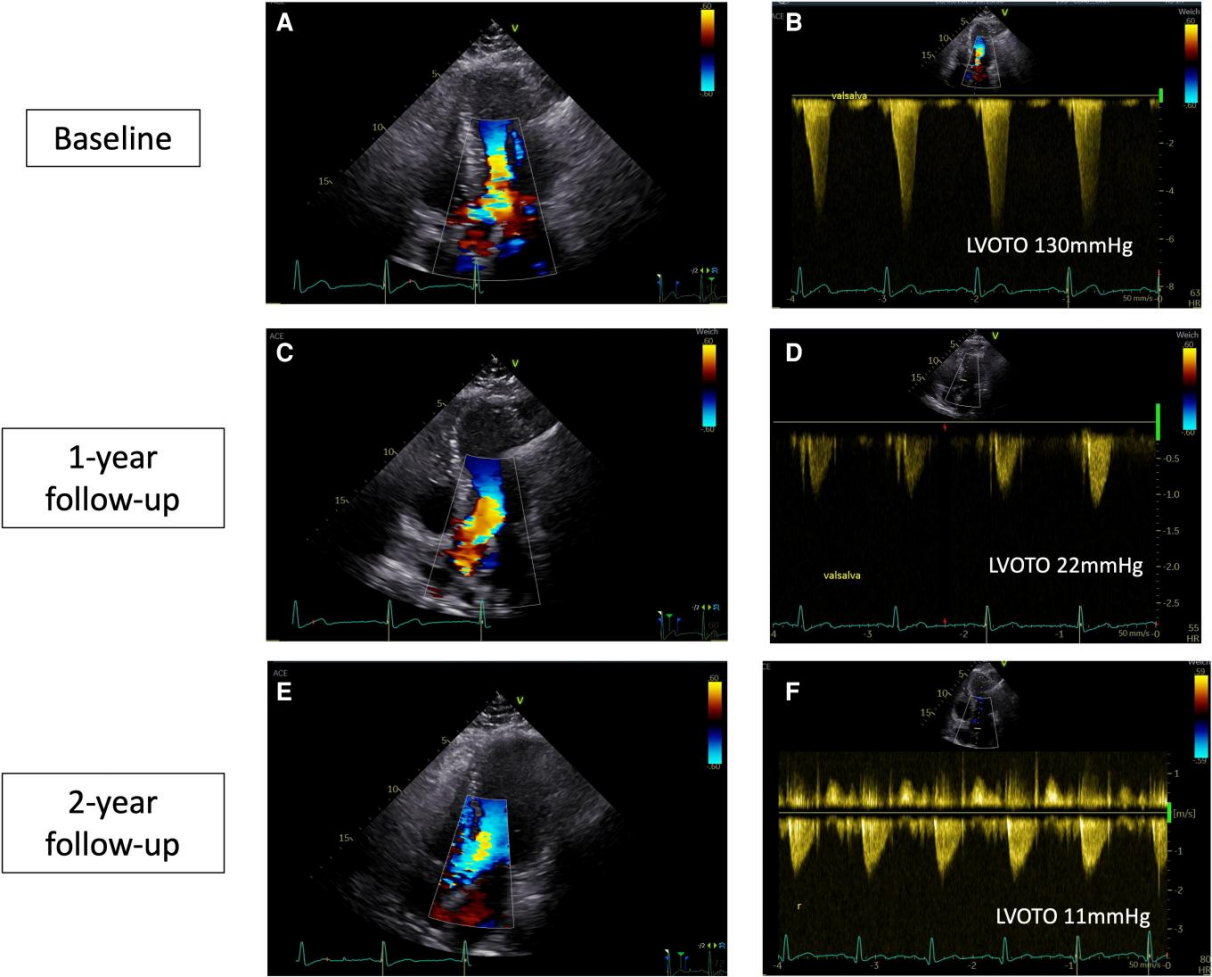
Functional effect on Valsalva left ventricular outflow graft obstruction under mavacamten shown in serial transthoracic echocardiography examinations. Colour Doppler in four-chamber view (A, C, and E) and continuous wave Doppler images (B, D, and F) are depicted. LVOTO = left ventricular outflow graft obstruction.
